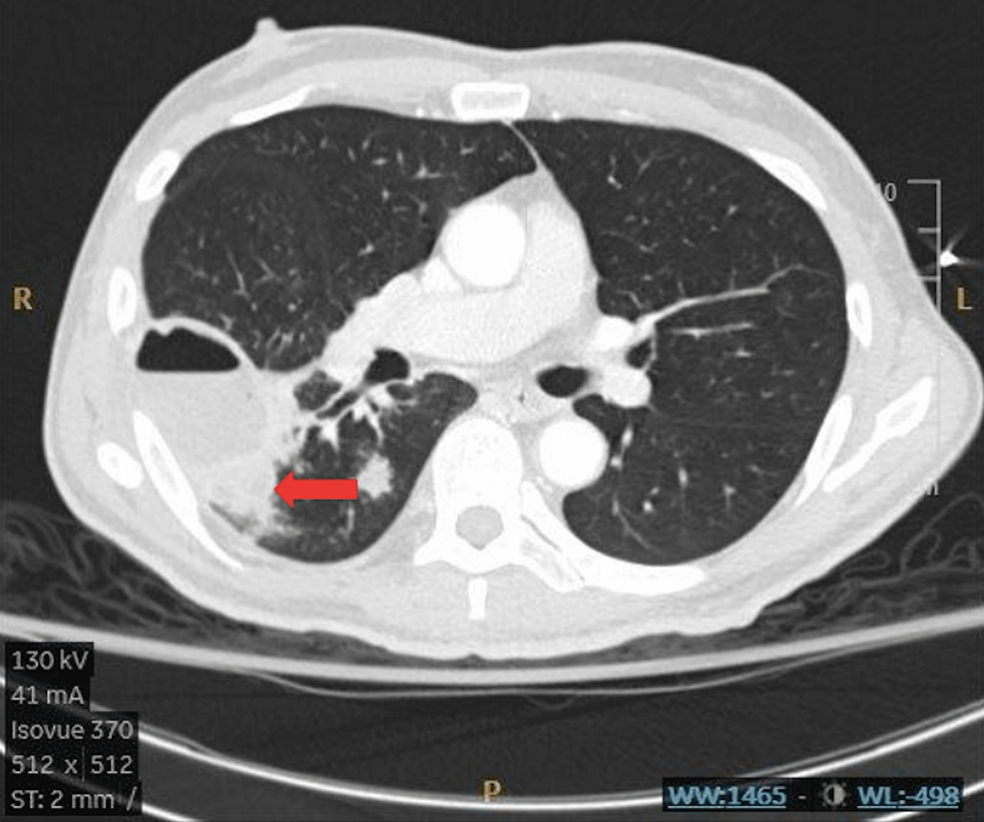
Axial chest CT showing complicated abscess. The red arrow point to the area of loculation.
